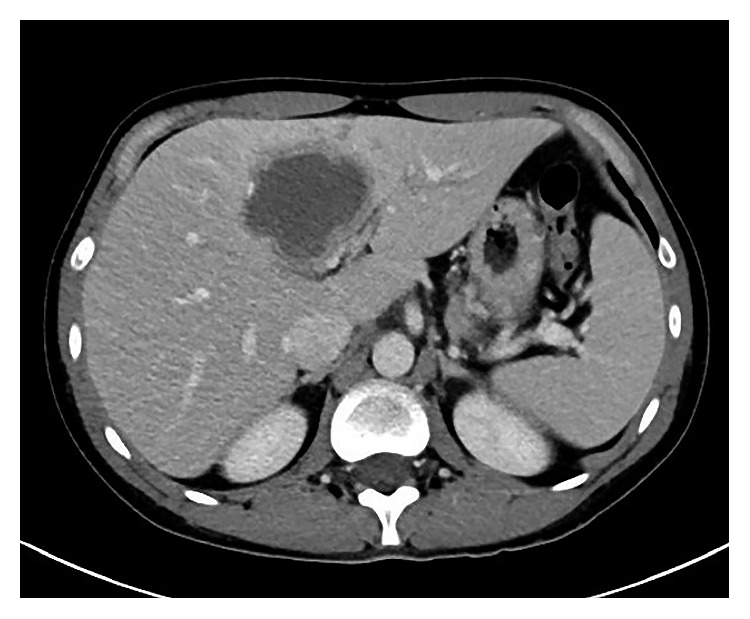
Abdominal CT scan on hospital admission revealing a complex lower attenuating 6.0 cm hepatic segment 4A/B lesion with a thick marginal rim, adjacent parenchymal inflammation, and biliary prominence.
